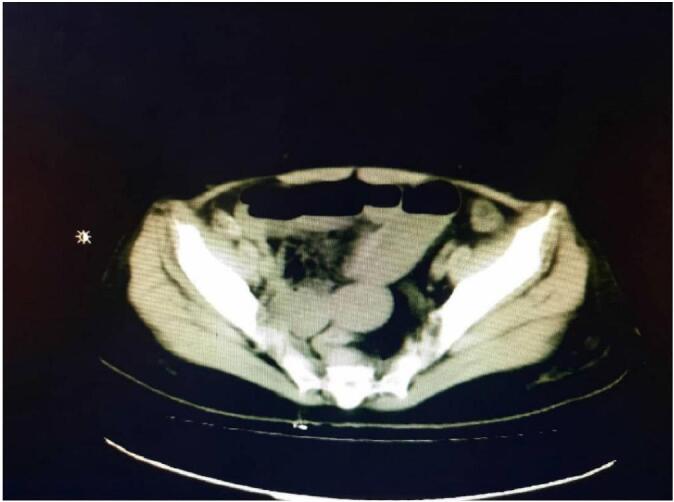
A CT scan image showing Dilated intestinal loops with aerobic fluid levels.
